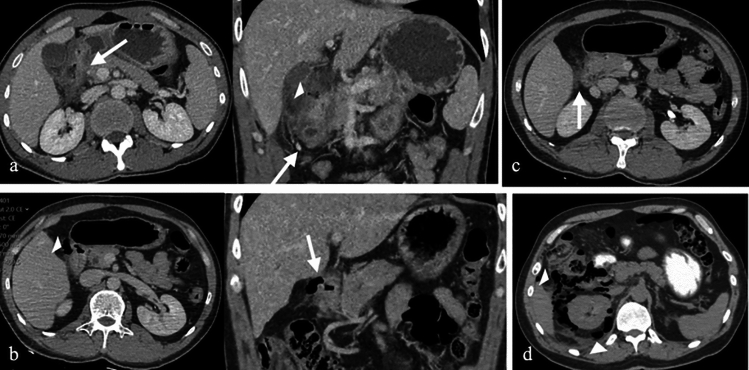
CT images from four different patients with duodenal perforation: a. Axial and b. Coronal IV contrast-enhanced CT images of a 34-year-old female and a 44-year-old male patient with duodenal ulcer perforations, showing diffuse duodenal wall thickening, periduodenal fat stranding, and free intra-abdominal air (arrows and arrowheads). c. Axial IV contrast-enhanced CT image of a 23-year-old female patient with post-traumatic duodenal perforation. d. Axial IV contrast-enhanced CT image of a 37-year-old male patient with duodenal perforation due to ERCP complications, demonstrating extensive retroperitoneal and periduodenal free air densities (arrowheads)
